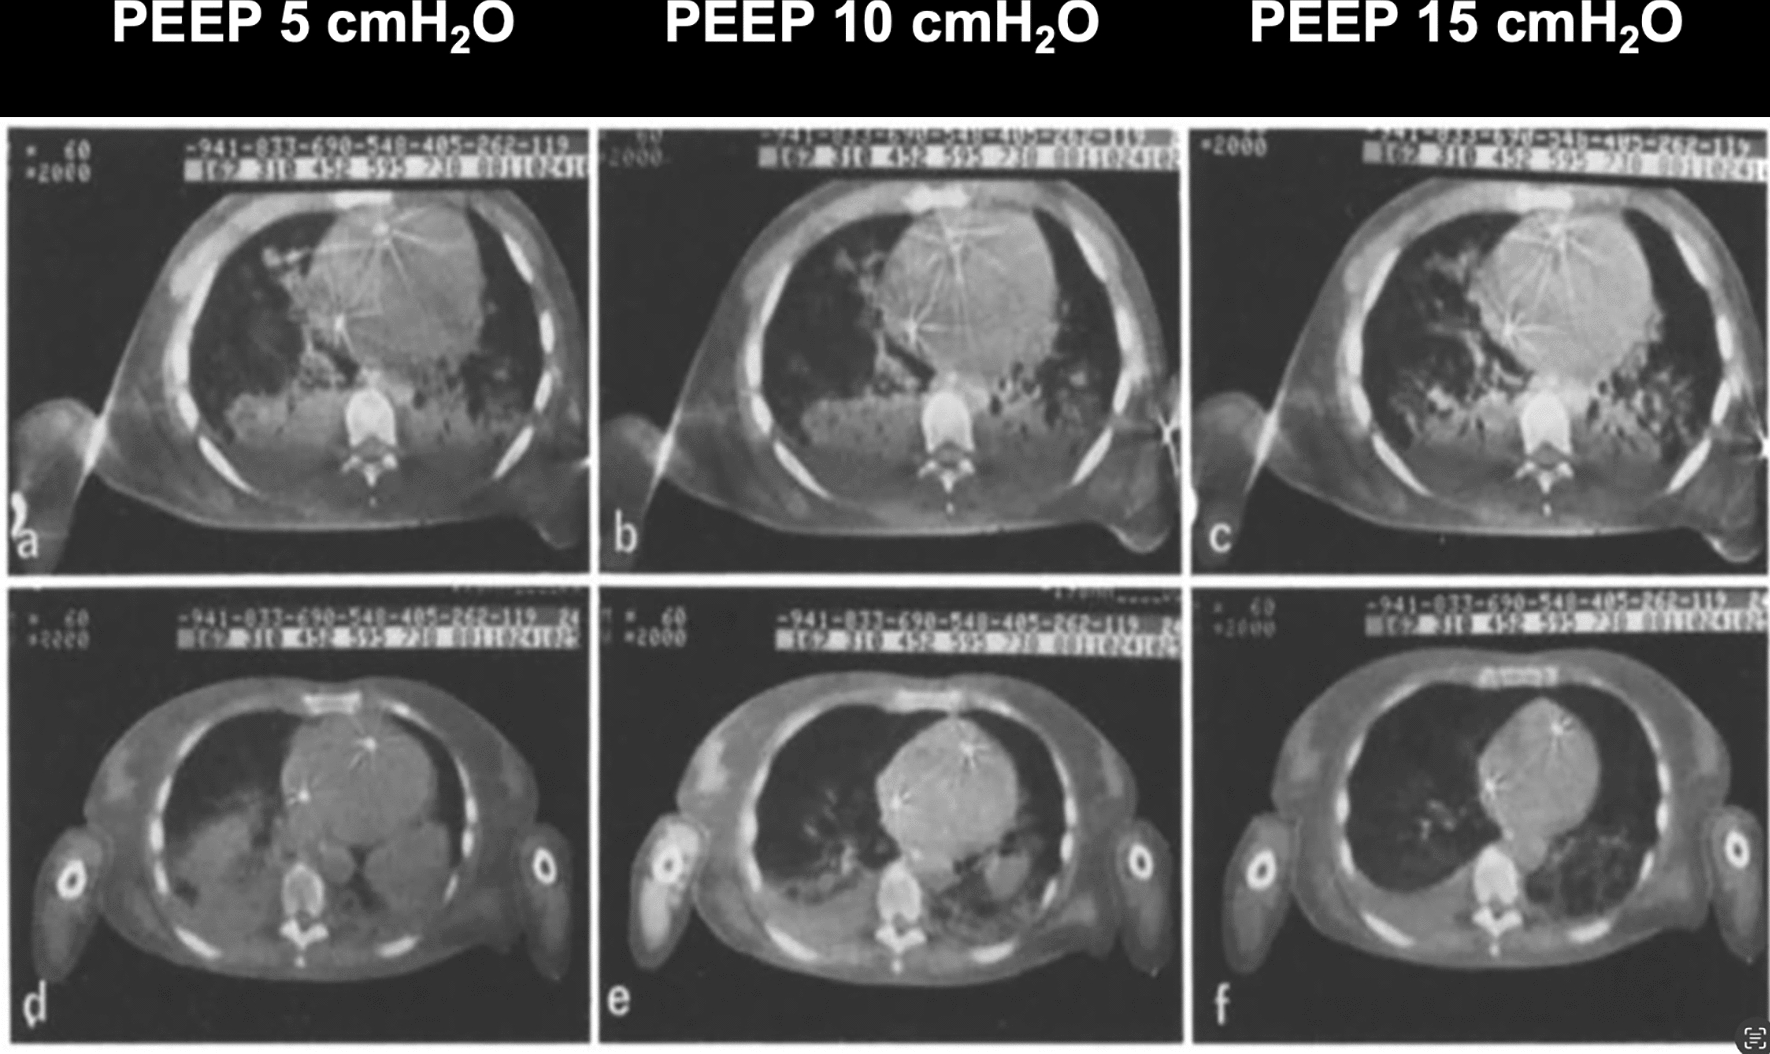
CT scan of the base at 5, 10 and 15 cmH_2_O. Upper line a–c Acute respiratory failure caused by bacterial pneumonia. The three different CT scans at varying level of PEEP show no substantial differences, no recruitment leading to the risk of overdistention. Lower line d–f Acute respiratory failure cause by sepsis. The densities are markedly reduced with the increase of the PEEP, meaning higher recruitment. Rearranged by Gattinoni et al. [13]
